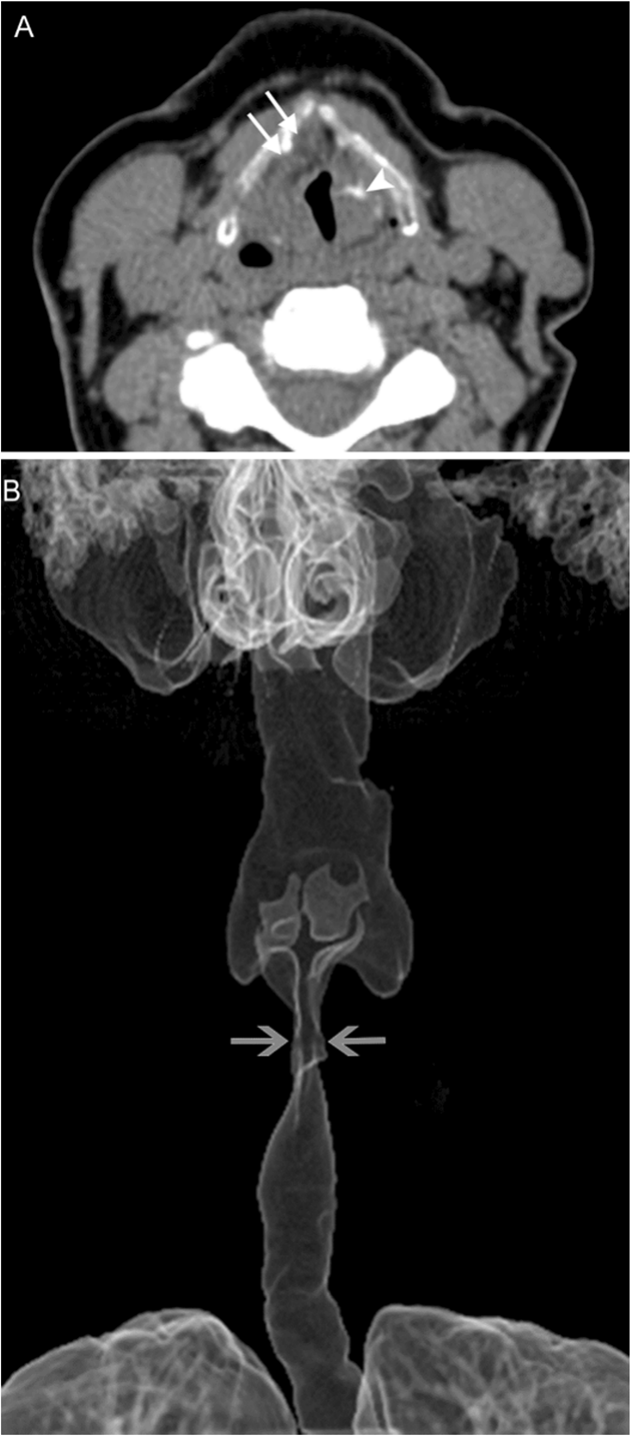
Laryngeal amyloidosis. A 69-year-old woman with recurrent laryngeal amyloidosis despite multiple interventions. Axial CT image (a) reveals marked soft tissue thickening of the laryngeal structures extending into the right pre/paraglottic space (white arrows) representing amyloid infiltration, and dystrophic calcification (white arrowhead) representing post-treatment change. The true vocal cords are not identifiable. 3D surface volume rendering (b) demonstrates marked narrowing of the glottic airway (gray arrows)
